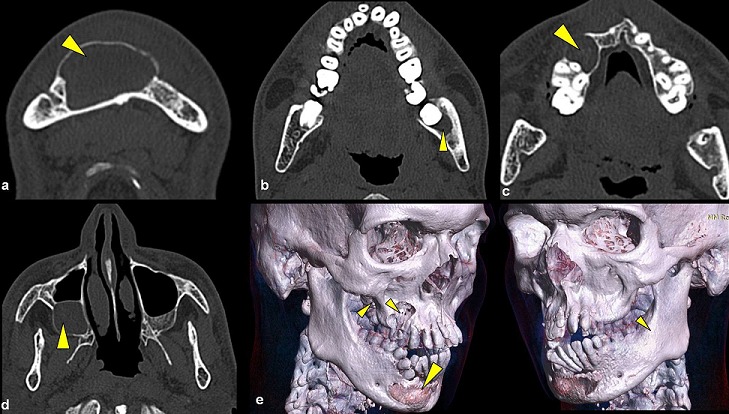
(a,b,c,d) transverse slides of CT, Formatted 3 dimensional images; e) showing multiple jaw cyst
